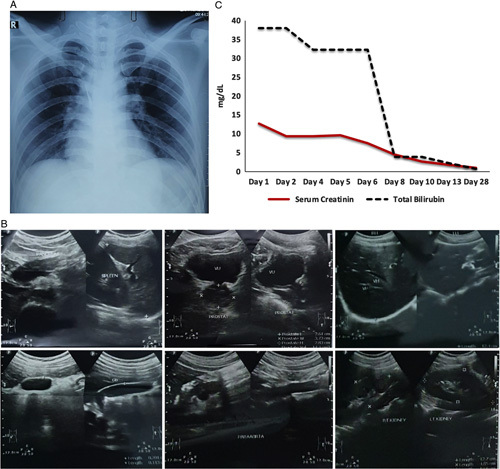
Chest radiograph examination showed: a thickening of the left hilum, and the heart abnormalities were absent (A). The results of the upper and lower abdominal ultrasound examination showed normal limits (B). Improvement of serum creatinine and total bilirubin levels of the patient (C).
